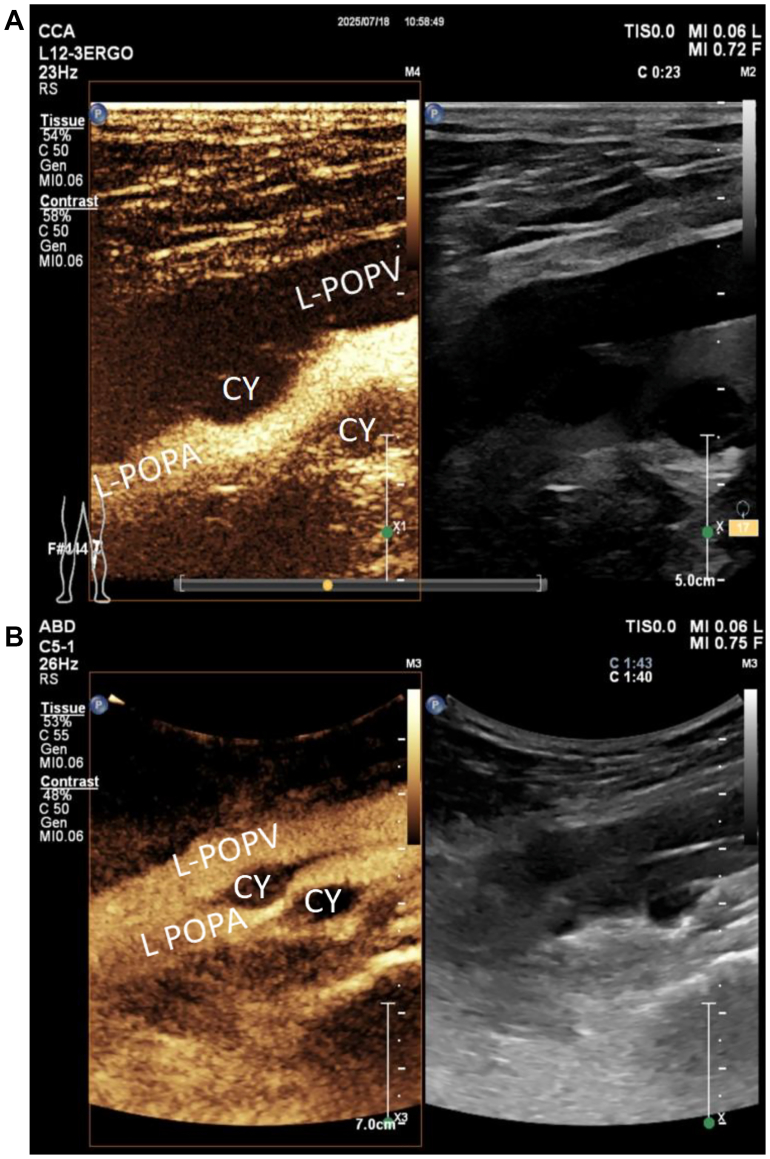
(A) Contrast-enhanced ultrasound (CEUS) scan from the first exercise cycle showed the left popliteal artery (*L-POPA*) was compressed by the popliteal cyst (*CY*) during the arterial phase, resulting in mild stenosis of the lumen. (B) CEUS scan from the third exercise cycle showed that the popliteal artery was compressed by the popliteal cyst, resulting in severe stenosis of the lumen during the venous phase. *L-POPV*, Left popliteal vein.
